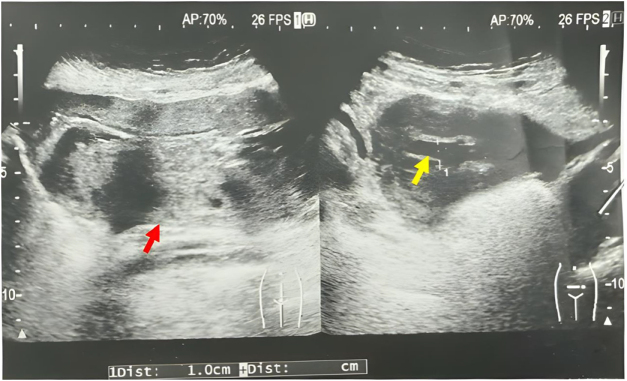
Transabdominal ultrasound demonstrated a normal-appearing uterus (red arrow) with a small amount of fluid in the endometrial cavity (yellow arrow). No free intraperitoneal fluid was detected.
